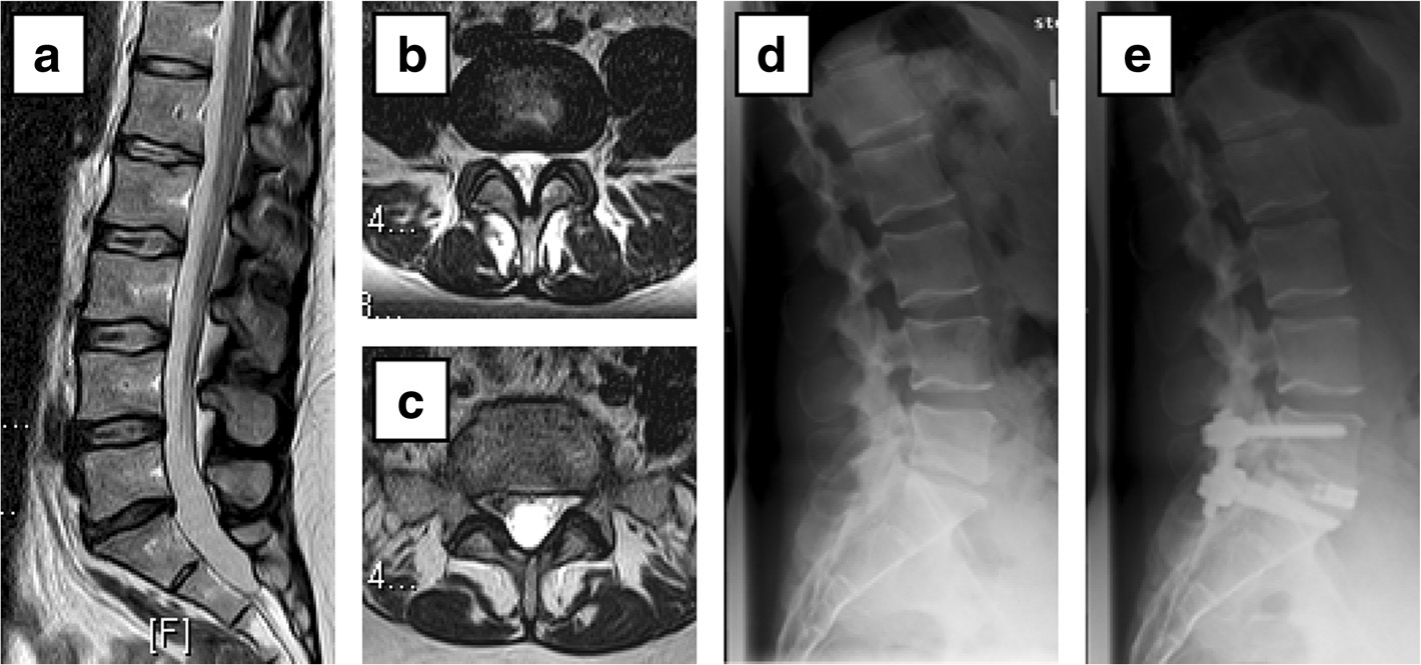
Confirmation of an uncertain indication for surgery. a-c T2-weighted magnetic resonance images preoperatively: a lumbar sagittal image and b axial lumbar image of L4/5 and c L5/S1. d Lateral radiographic image preoperatively and e postoperatively. The patient presented with chronic lumbar back and sciatic pain on the right side. Moderate intervertebral disc degeneration was observed between L5 and S1 (Pfirrmann grade 3-4 [13]) and a secondary neuroforaminal stenosis on the right side. Moderate facet joint degeneration was seen at L4/5 (Weishaupt [14, 15] grade 1 right and grade 2 left facet) and L5/S1 (Weishaupt grade 1 right, grade 2 left facet). Recommendation before inpatient gradual diagnostics (IGD): lumbar fusion of L5/S1 with transforaminal lumbar interbody fusion (TLIF). Results obtained during IGD: • epidural injection of L5/S1: 20 % sciatic pain relief. • bilateral facet joint infiltration of L5/S1: 80 % back pain relief. • bilateral sacroiliac joint infiltration: 10 % back pain relief. • iliac crest infiltration at muscular insertion of erector spinae: 80 % pain relief. Recommendation after IGD: lumbar fusion of L5/S1 with TLIF. At 1 year follow-up, the patient reported no pain
